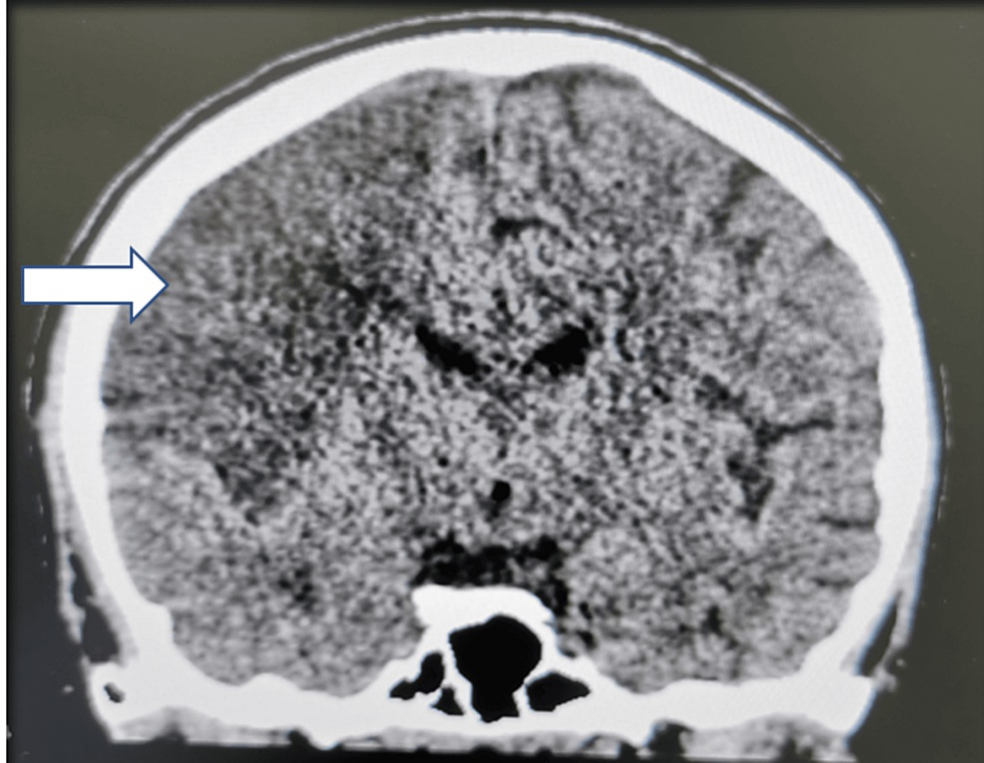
MRI brain in Case 3 showed that the patient developed right ischemic infarction of middle cerebral artery.
